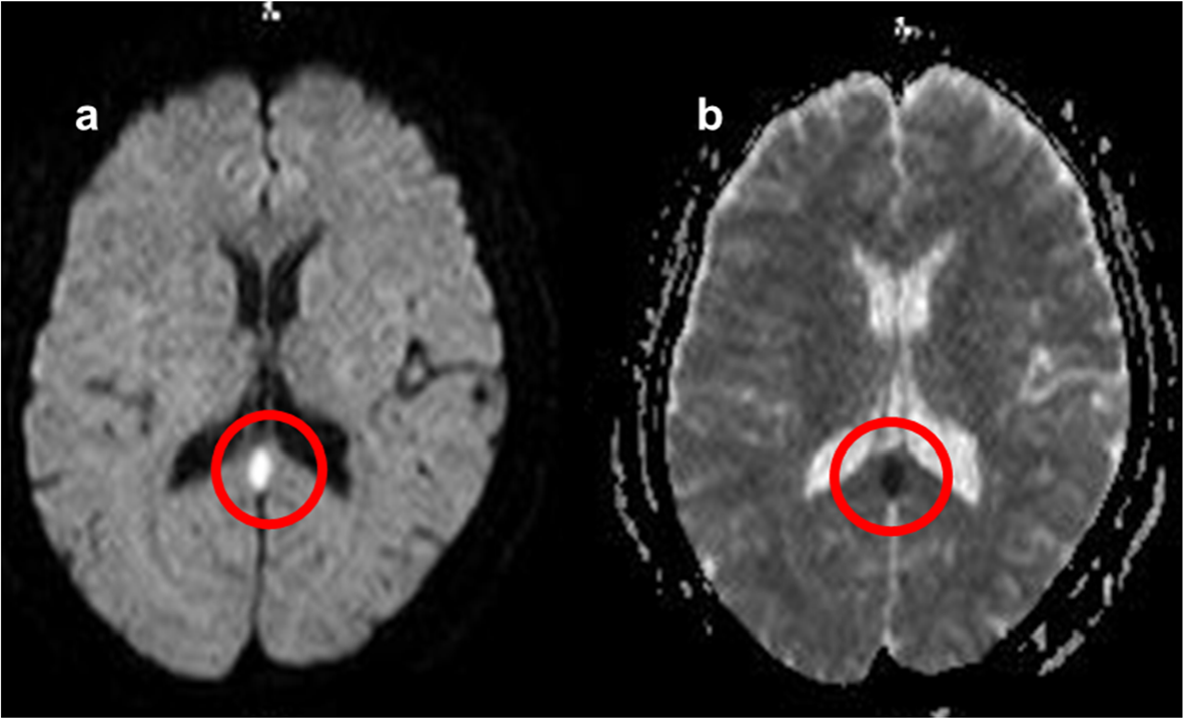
Head magnetic resonance imaging on admission day 2. a Diffusion-weighted imaging (DWI), b Apparent diffusion coefficient (ADC). Head magnetic resonance imaging revealed an ovoid lesion in the central portion of the splenium of the corpus callosum. The lesion appears as reduced diffusion on DWI and has a low ADC value (red circles)
